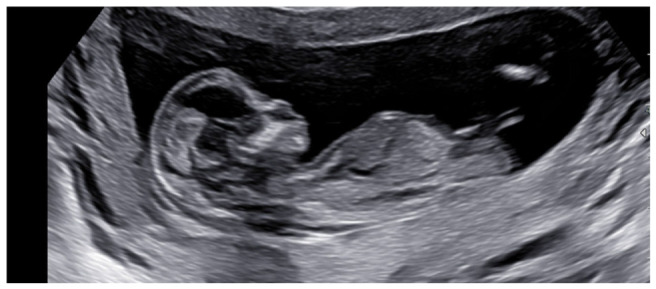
Normal first-trimester ultrasound.
